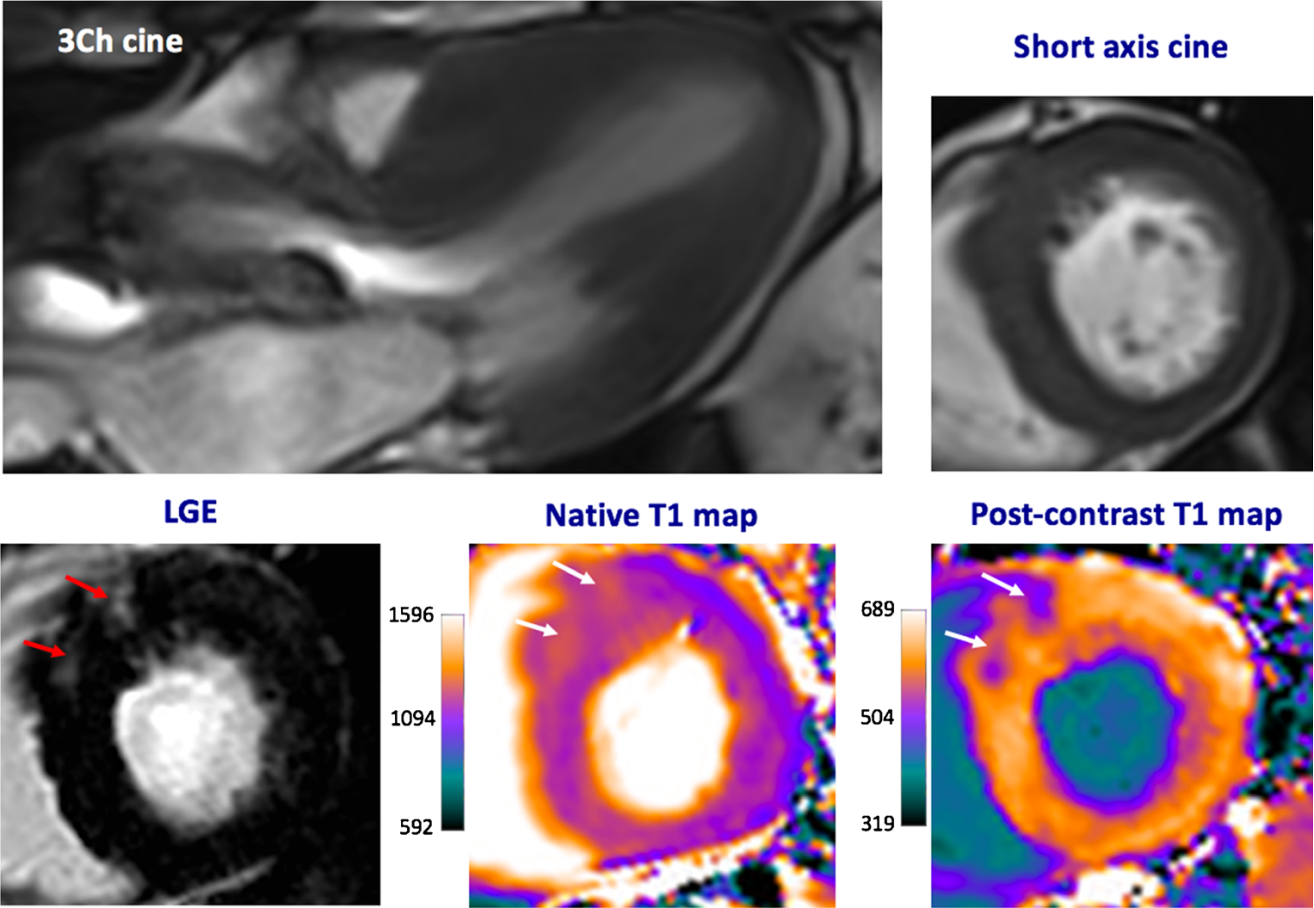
Cardiac magnetic resonance imaging in a patient with severe aortic stenosis. Predominant asymmetrical hypertrophy of the anteroseptum is seen with associated patchy mid-wall late gadolinium enhancement (LGE, *red arrows*). These areas are also identified visually using native and post-contrast T1 maps (*white arrows*)
